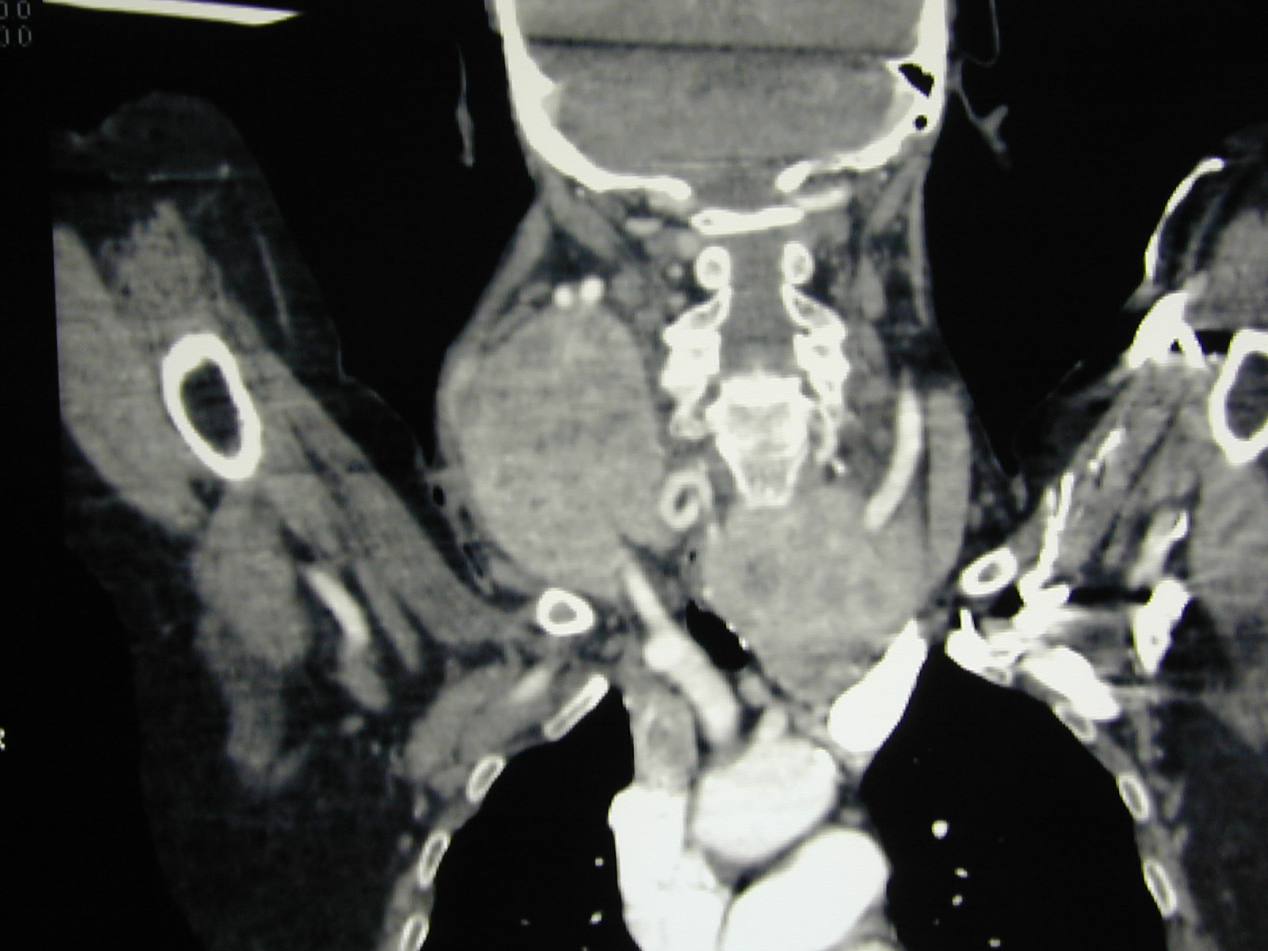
Contrast enhanced CT scan, coronal reconstructed image. A thyroid mass extending from the submandibular and submental regions to the parapharyngeal space and superior mediastinum is evident.
